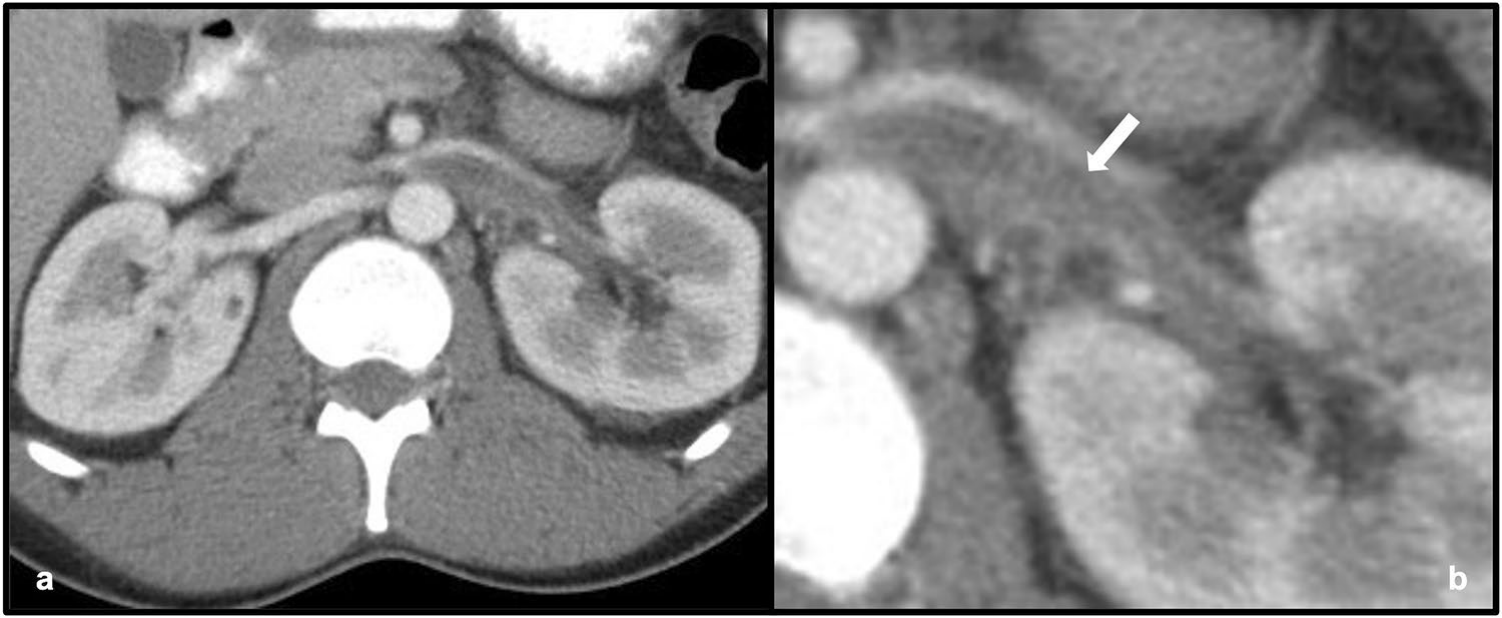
35-year-old male who presented with abdominal pain, found to have lupus nephritis on renal-biopsy. Axial wide (a) and narrow (b) field-of-view images of a contrast-enhanced CT demonstrate a filling defect within an expanded left renal vein (white arrow), consistent with acute renal vein thrombosis. The normal right renal vein is shown for comparison
